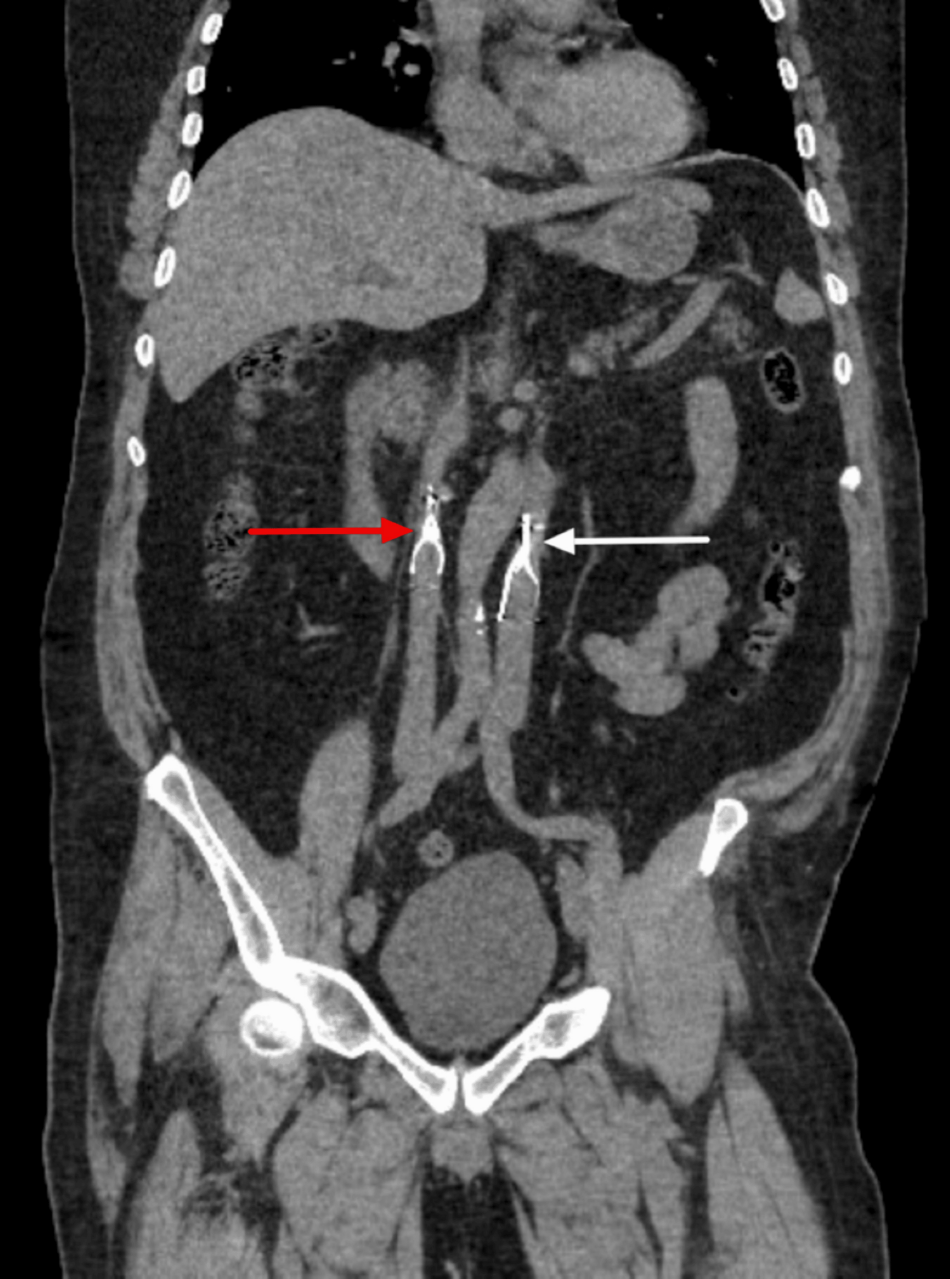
Coronal CT demonstrates a filter in the right IVC (red arrow) and the left IVC (white arrow). CT, Computed tomography; IVC, Inferior vena cava
